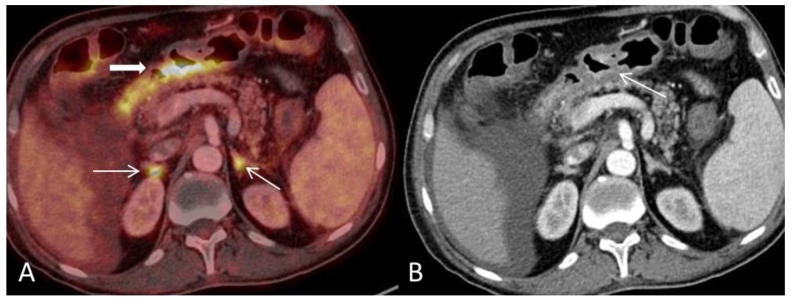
(A) Axial PET and CT fused image shows pathological FDG-uptake in the wall of antrum and to a lesser degree in pylorus (bold arrow). Both adrenal glands are seen with high FDG-uptake with no morhological changes, the foci were intepreted as physiological and most likely stress-related (arrows); (B) Axial CT shows thickening of the antrum wall with mucosal enhancement (arrow). Ascites surrounding the liver is also present.
